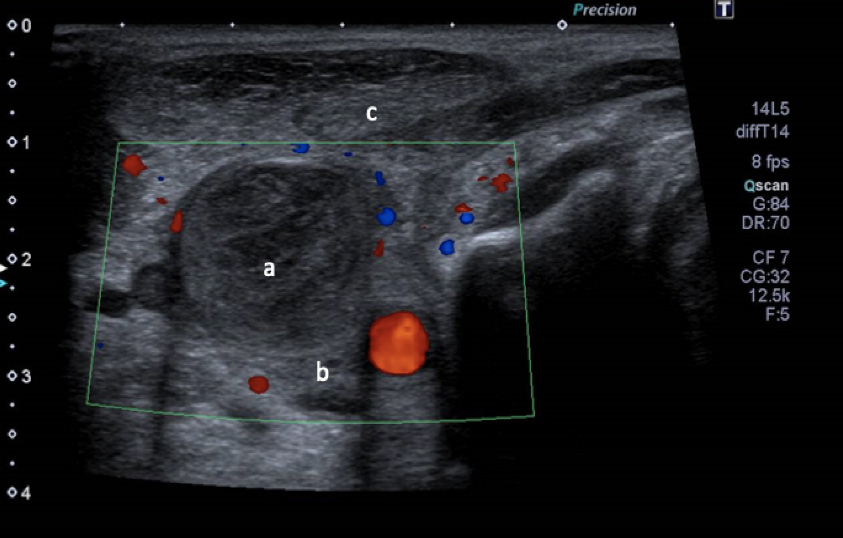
Ultrasound Doppler of the right cervical region performed on 6 November 2019: signs of an enlarged right internal jugular vein with heterogeneous echogenic material (a) over its entire height and taken over by collaterals, which led to the diagnosis of a recent thrombosis of the right internal jugular vein; signs of an infiltration and thickening of soft cervical tissue (b); numerous right cervical infracentimetric lymph nodes; no abscess of the soft parts of the sterno-cleido-mastoid muscle (c) was observed.
